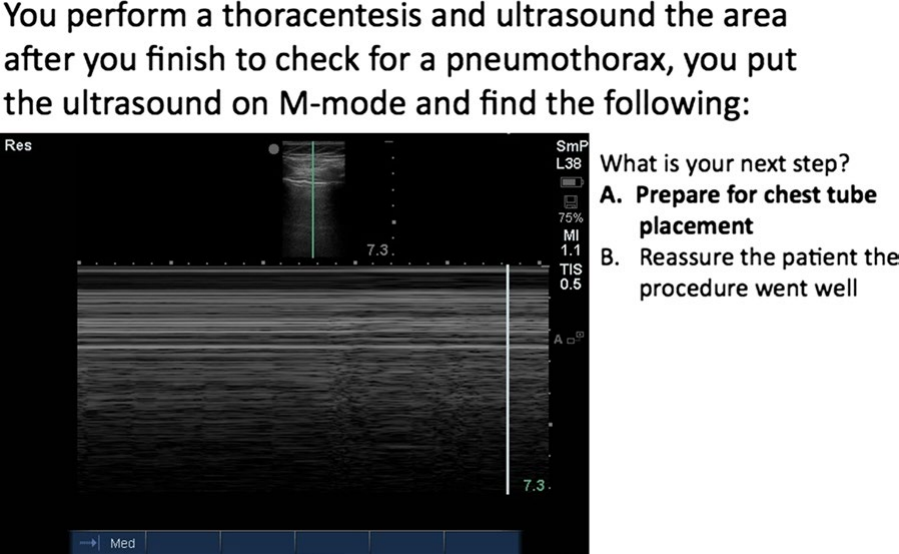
Sample test question of clinical application of bedside ultrasound
